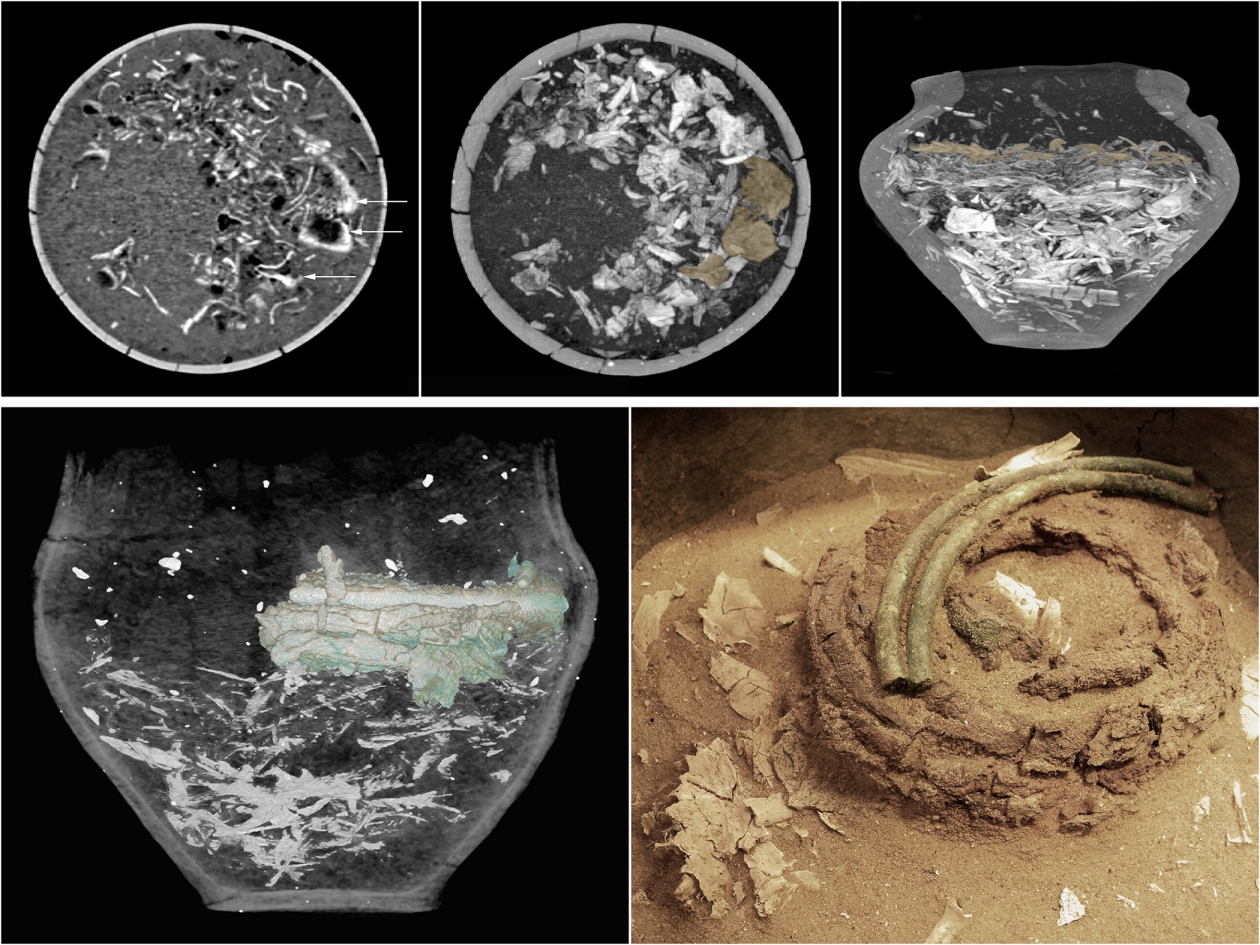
The results of CT imaging of the fills of the urn from graves 557 and 528. In the upper row, fragments of the skull vault bones are visible in the upper part of the fill of the urn from grave 557; the arrows and the brown colour mark the identifiable fragments of the parietal bones and the right zygomatic bone. In the lower row, the urn from grave 528 and the visualization of metal objects in MIP reconstruction and their actual appearance during the exploration.
